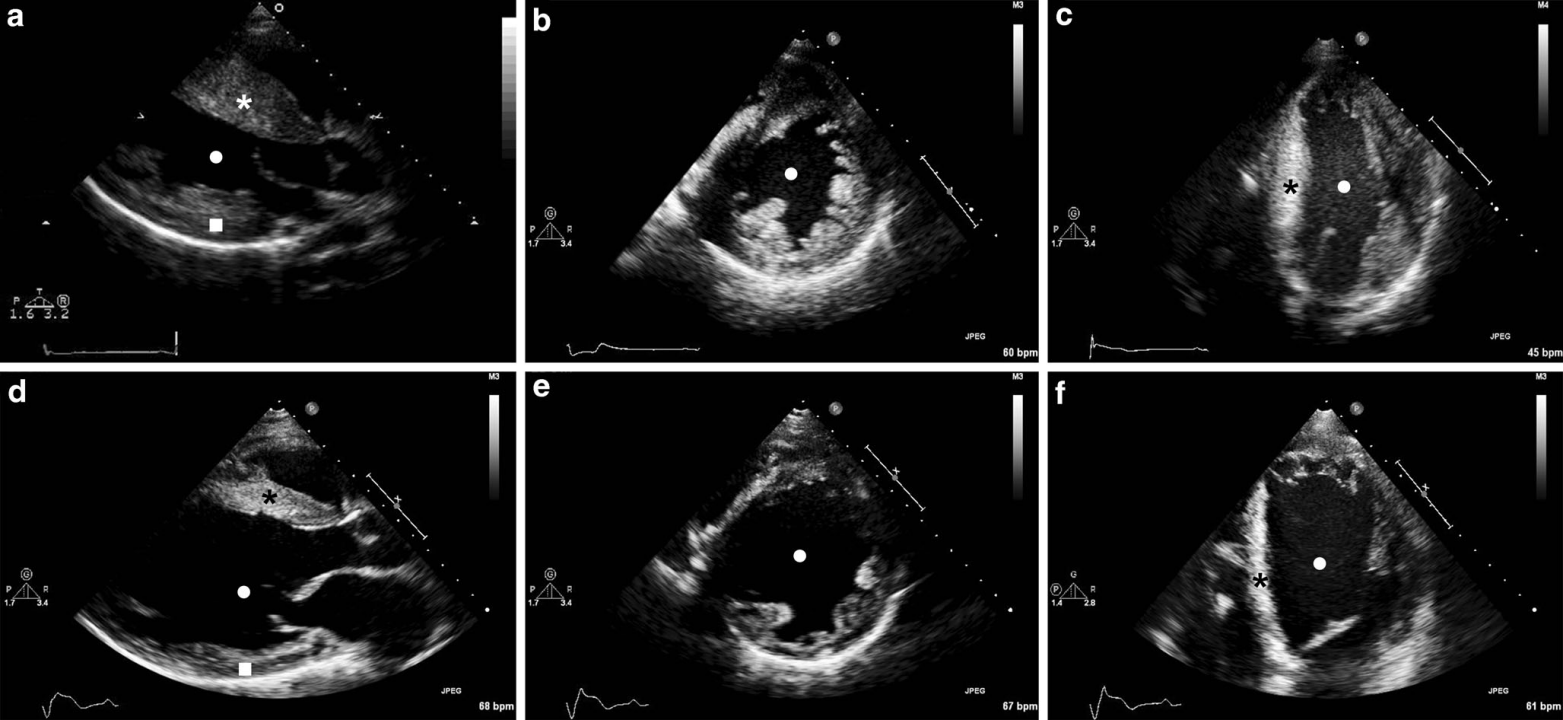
Echocardiographic follow-up of case 1. Transthoracic echocardiogram of case 1 in 2005 (a–c) and 2015 (d–f). During 10 years of follow-up there was a gradual decrease of septal (*) and left ventricular posterior wall (▪) thickness and an increase of left ventricular dimension (●)
